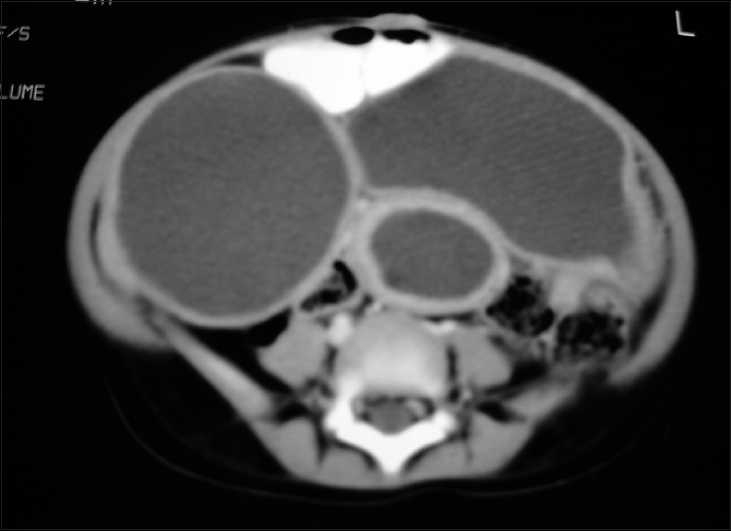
CT scan of the abdomen showing well-defined duplication cysts in the upper abdomen
